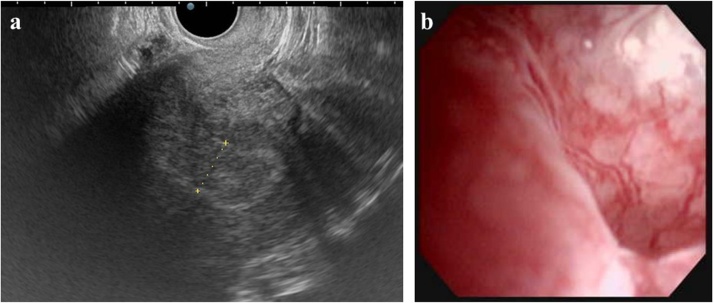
(a) Transvaginal ultrasonography: 20 mm of endometrium thickness (broken line). (b) Hysteroscopy: Tumor lesion with entire circumference of atypical vessel in corpus uteri.
